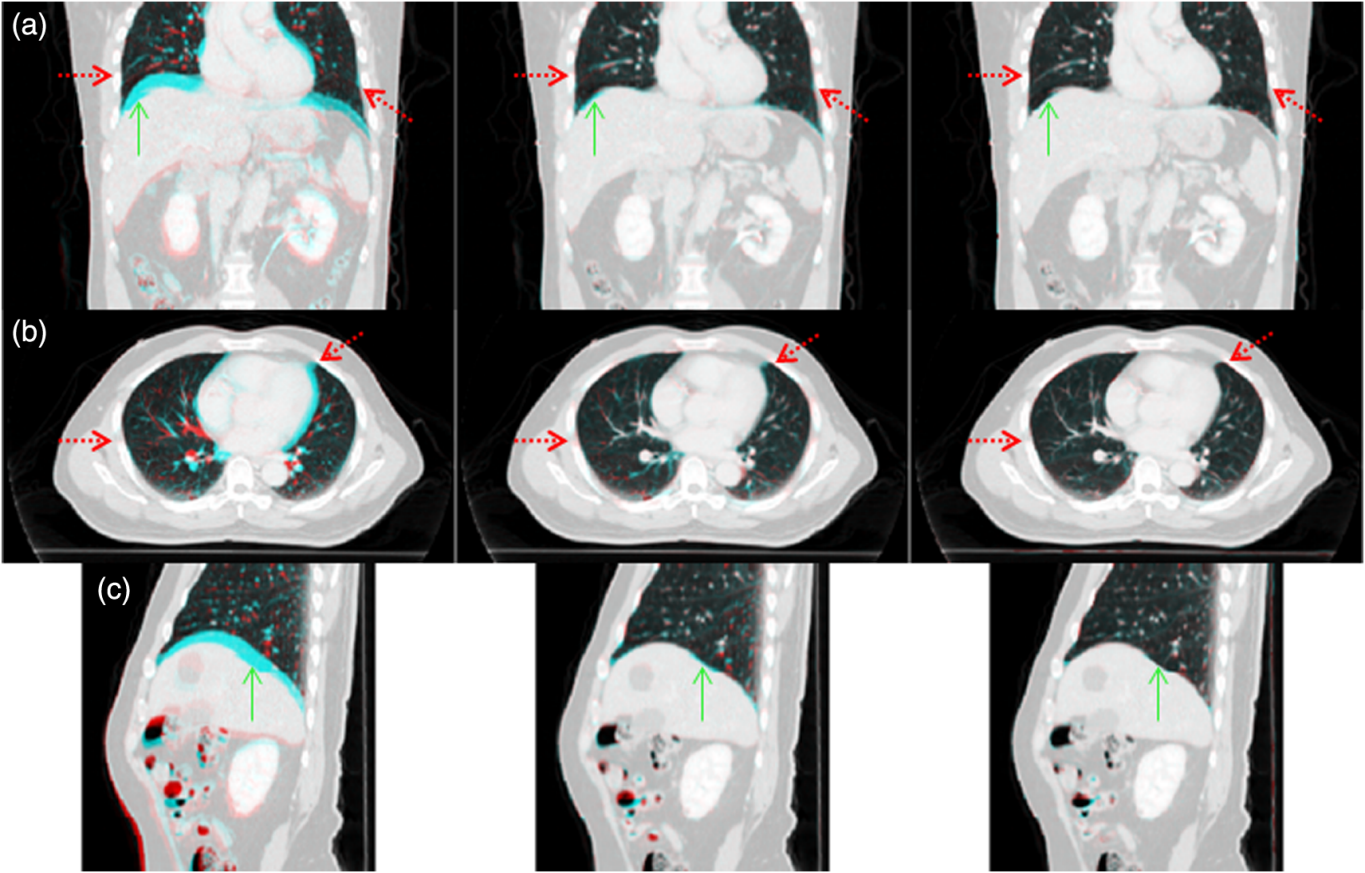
Main anatomical views of 3-D CT registration results for case #P0 of the liver dataset: (a) coronal, (b) axial, and (c) sagittal views for the color-coded (red-cyan) intensity differences between volume pair before registration (left), after registration using Demons with isotropic Gaussian kernel, iso-dem, (middle), and guided image filtering with random SLIC clustering, rdn-gif, (right). Registration using our method (right) improves registration accuracy especially close to the lung and liver surfaces (depicted by corresponding red dotted and green solid arrows, respectively).
